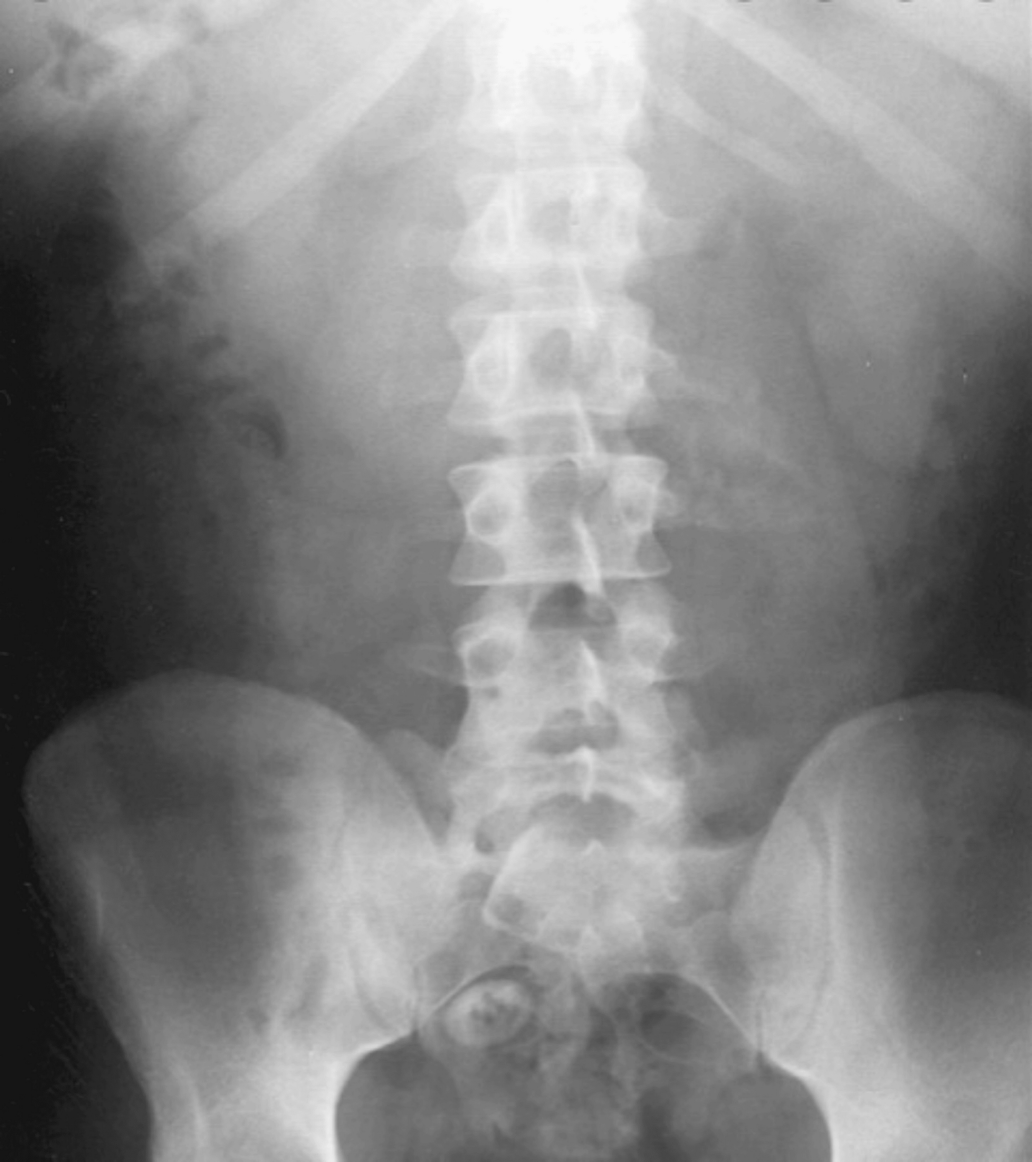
X-ray abdomen of the patient showing no obvious abnormal dilatation of the bowel loops or any free air
